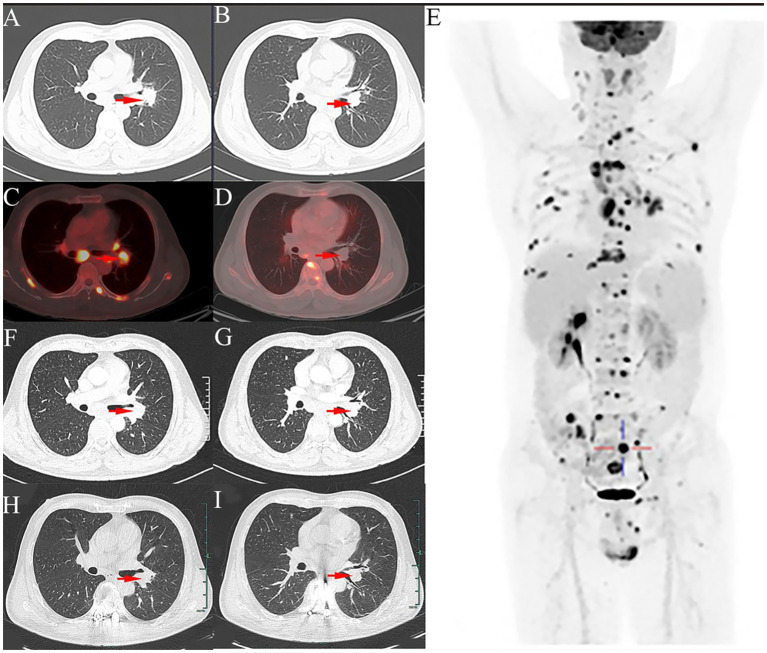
Dynamic radiologic changes of the left hilar/left upper-lobe mass and systemic FDG uptake during the clinical course (red arrows). (A, B), April 2025 (contrast-enhanced chest CT). (C, D), May 15, 2025 (^18F-FDG PET). (E), May 15, 2025 (^18F-FDG PET maximum intensity projection showing disseminated FDG-avid lesions). (F, G), July 2025. (H, I), January 2026.
